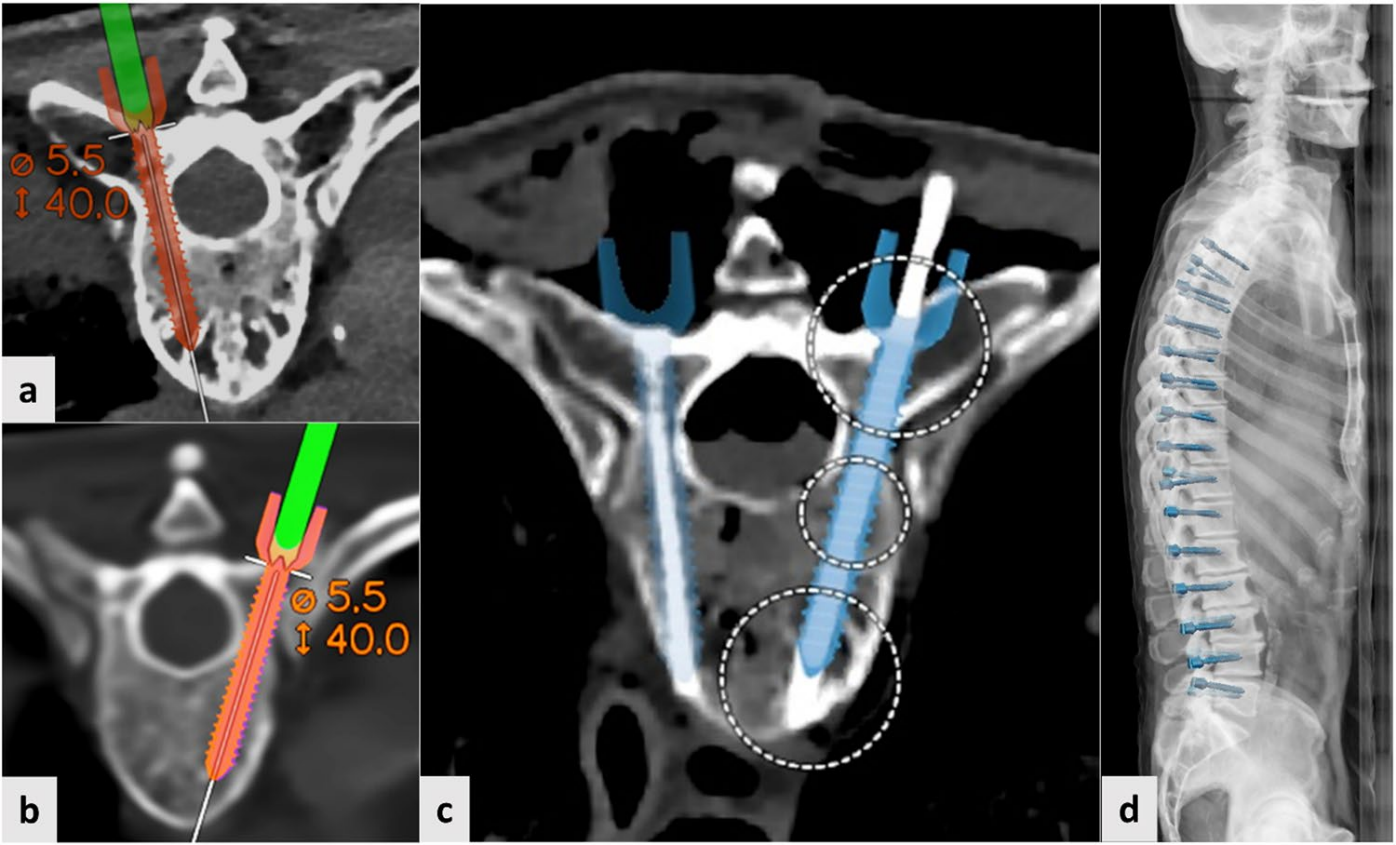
(a) Example of planned intraoperative screw position in thoracic vertebra on a CT-scan; (b) example of planned intraoperative screw position in thoracic vertebra on a sCT scan; (c) axial postoperative CT-scan with a virtual screw positioned over the k-wire. The dotted circles could be used to adapt the screw position to exactly overlay the k-wire (d) sagittal reconstruction of all postoperative screw positions in the navigation software
